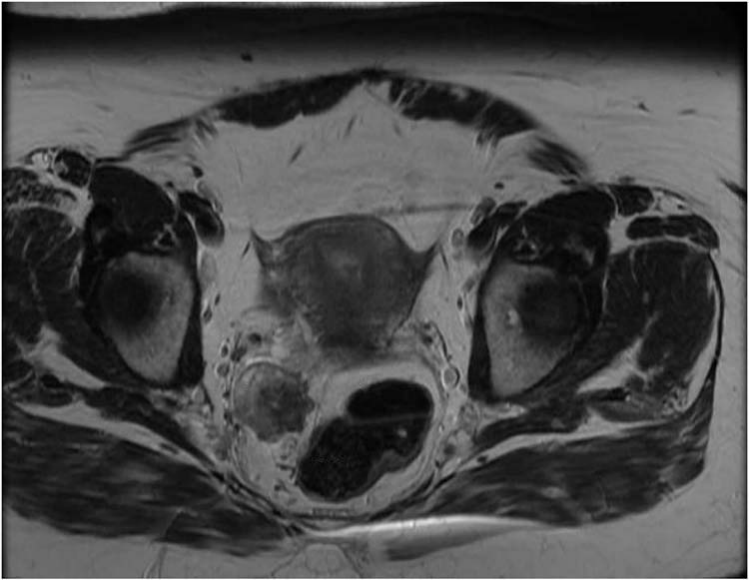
Latest pelvic MRI on initial consultation (T2-weighted images).
